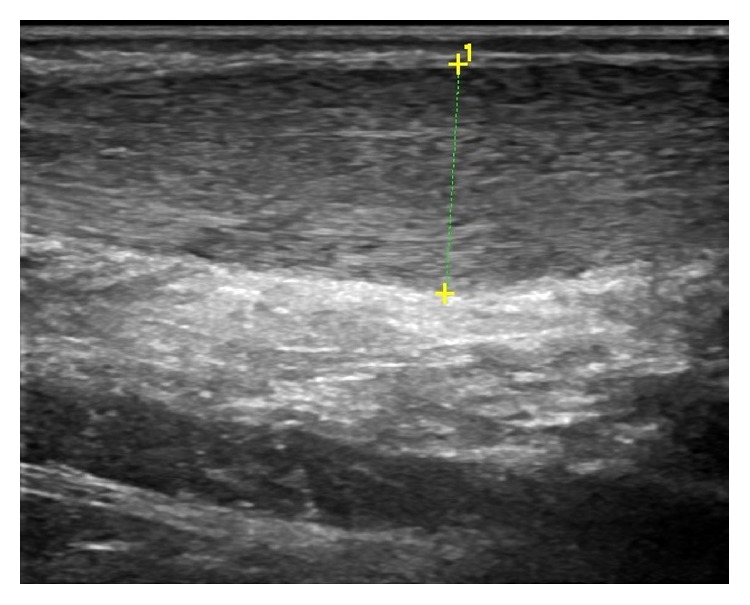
Ultrasound scan control of the Achilles tendon on 24-month follow-up.
